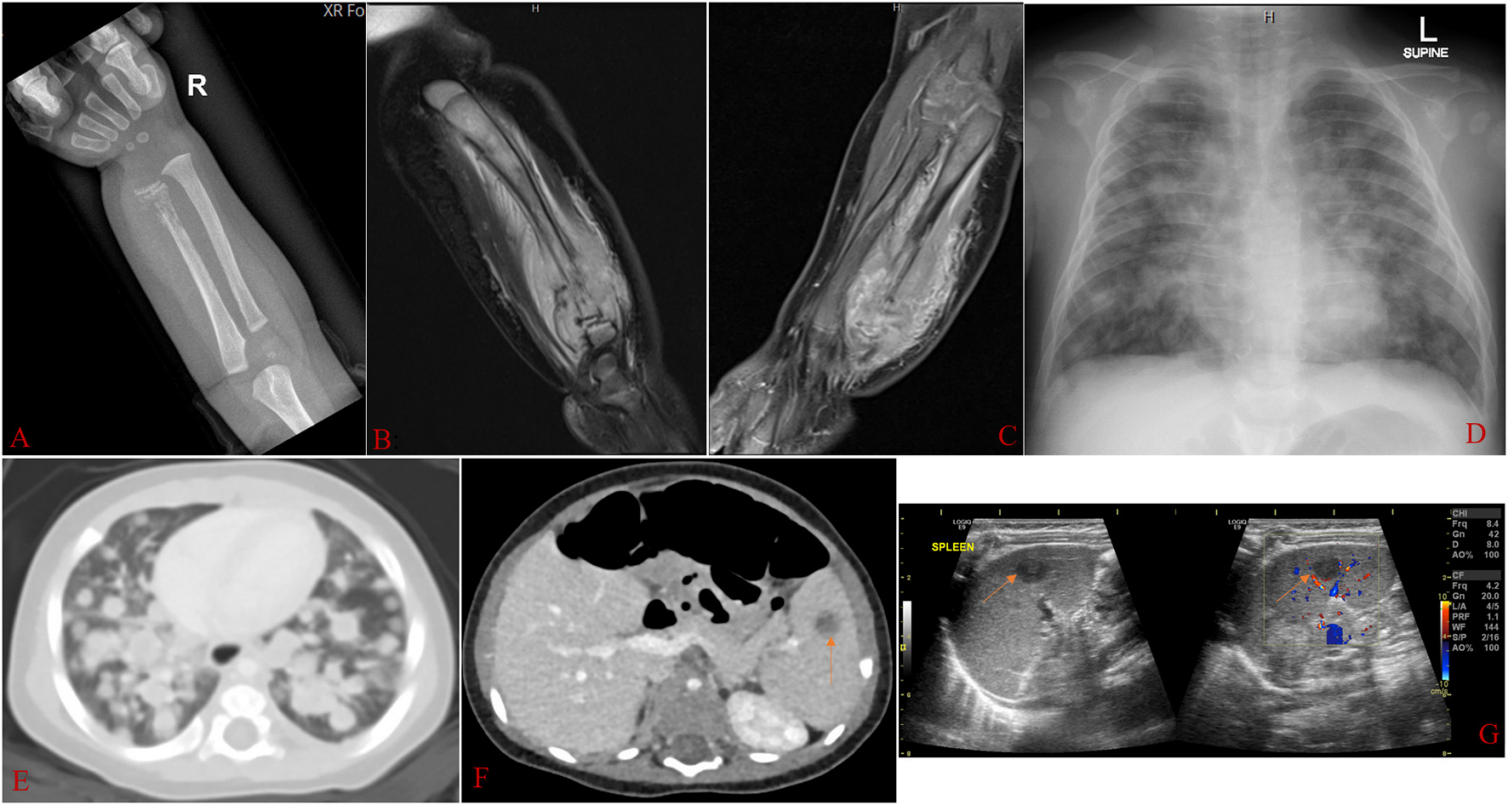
Radiographic images taken at about 3½ months of age. (A): Radiograph of the right forearm showing abnormal texture in the distal ulnar, with areas of bone destruction and resorption surrounded by soft tissue swelling. (B,C): MRI of the right forearm (with and without contrast) showing destructive bone lesion involving the ulnar with a large, enhancing soft tissue mass extending into the interosseous space, measuring 2.5 × 2.5 × 4.5 cm. The mass involves the diaphysis and distal metaphysis. There is periosteal reaction and edema in the surrounding soft tissue and subcutaneous fat. There is also abnormal signal and enhancement of the distal ulnar growth plate with displacement. (D,E): Chest radiograph and chest CT scan showing nodular lesions in both lungs. (F,G): Abdominal CT scan and ultrasound showing the right hepatic lobe exceeding the costal margin, measuring 8.6 cm craniocaudally. The spleen measures 7.0 cm in length and shows two focal hypodense lesions, the largest measuring 1.01 × 0.86 cm (arrowed).
